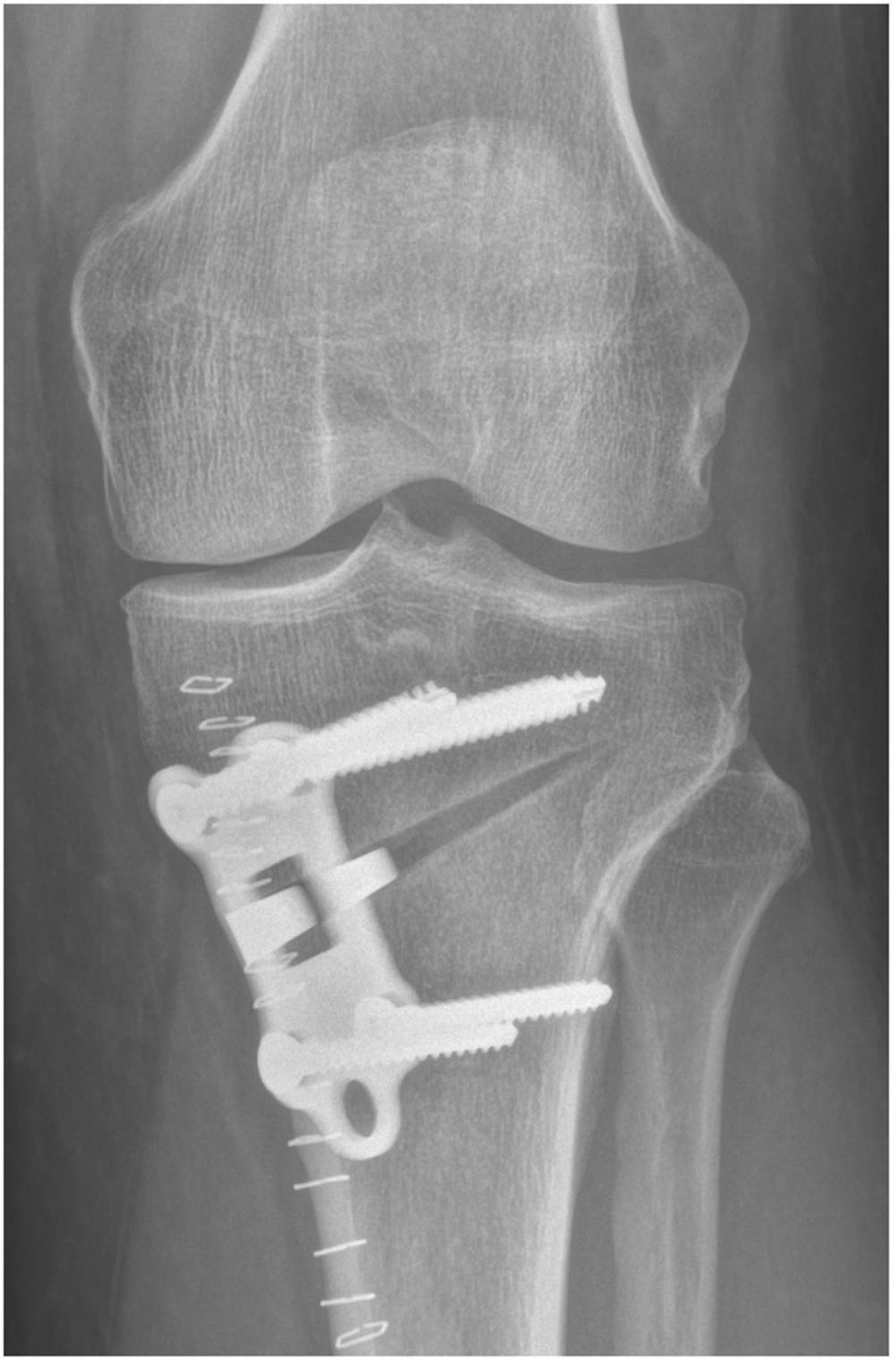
Postoperative radiograph.
